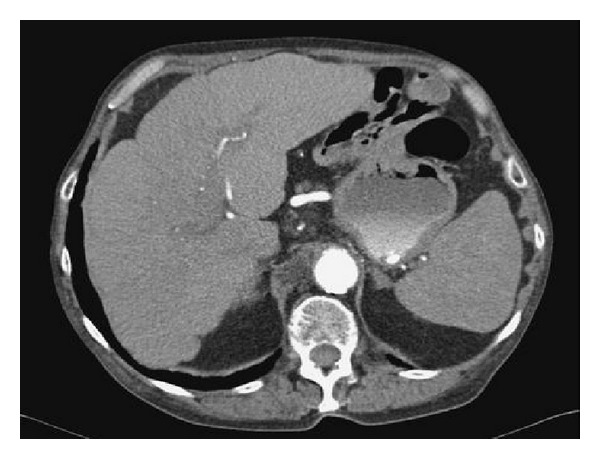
CT scan showing nodular liver contour, indicative of early hepatic injury and cirrhosis.
